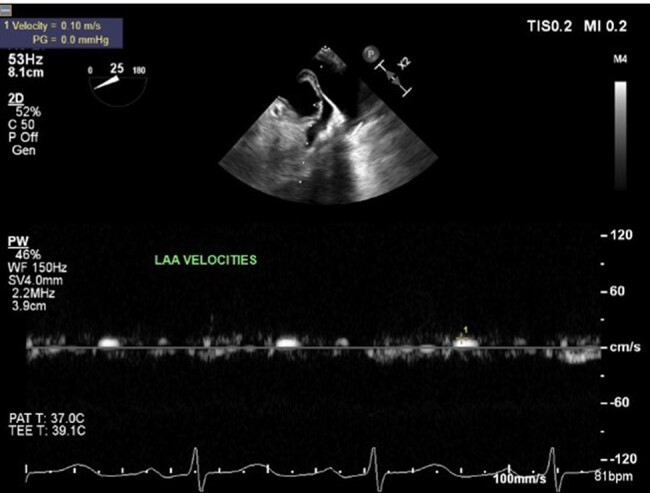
Low left atrial appendage emptying velocities (8 cm/s: *N* 60 ± 14 cm/s) indicating hypokinetic left atrial appendage (pulsed Doppler evaluation during transoesophageal echocardiogram).
